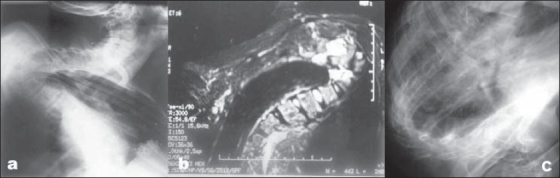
(a) Preoperative plain skiagram (b) Midsagittal T2-weighted MRI image of dorsal spine showing severe kyphoscoliosis with internal gibbus at the apex of the curve. (c) Postoperative skiagram taken six months after surgery shows gap grafting and correction of kyphosis.
